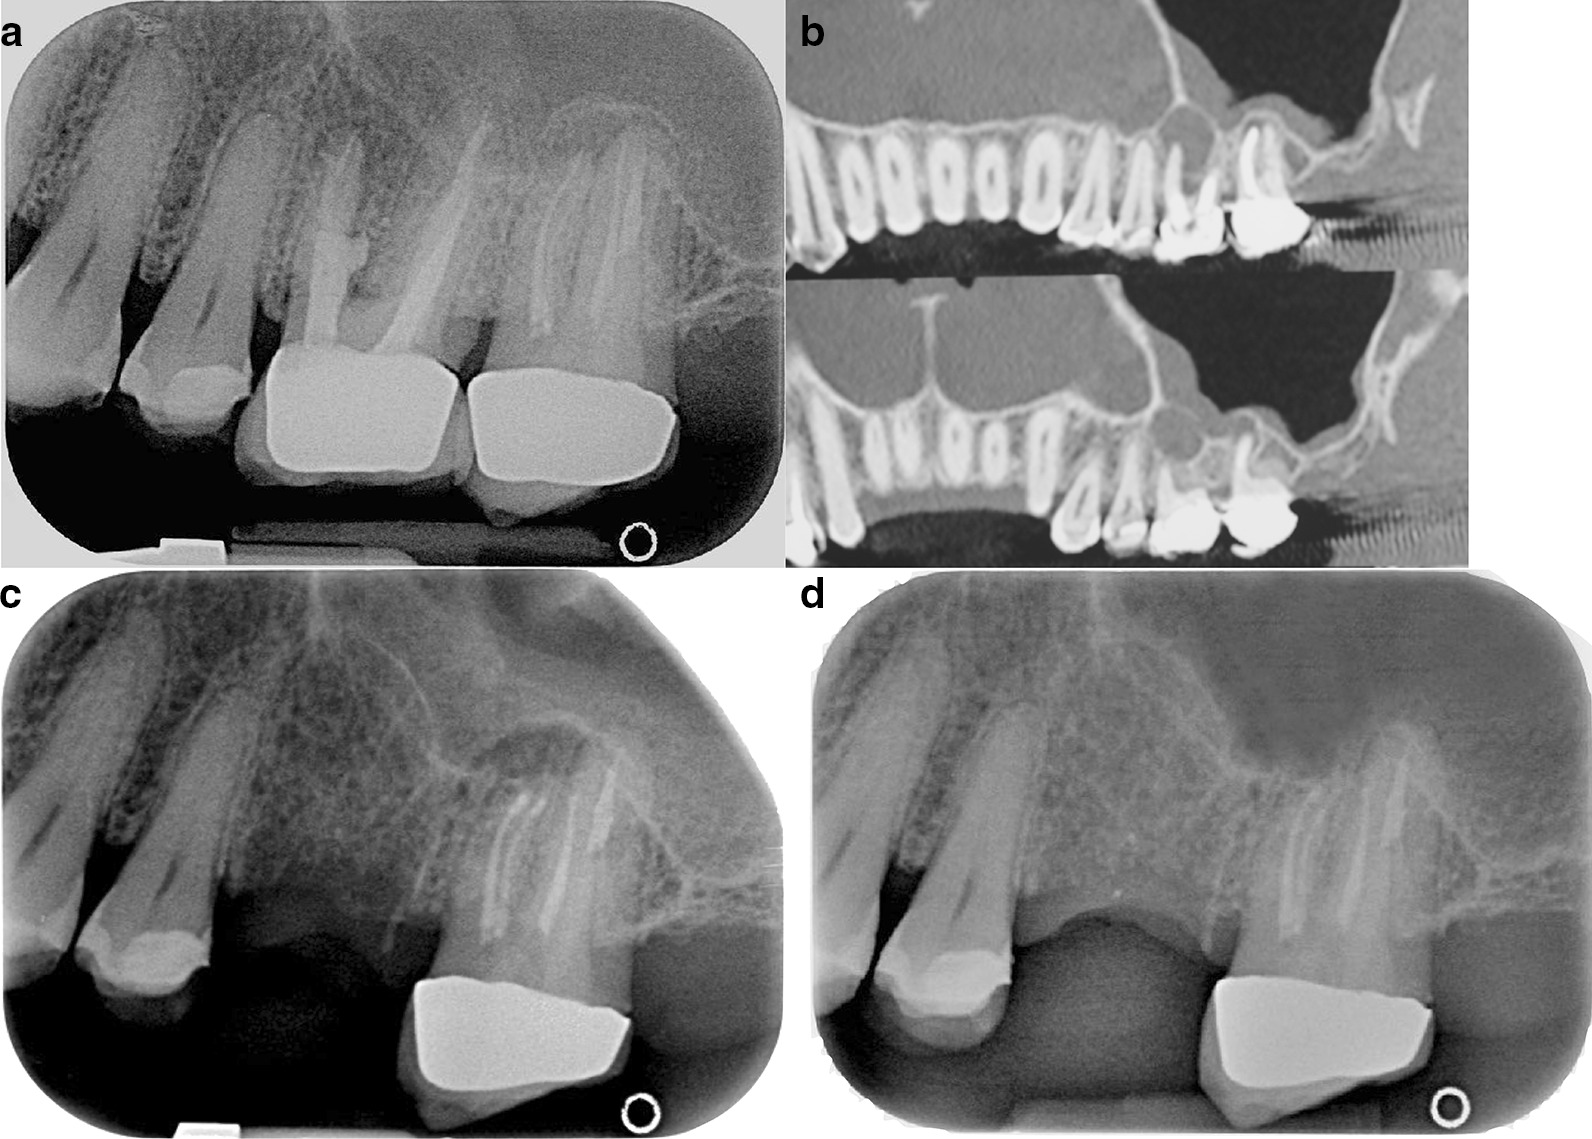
a Pre-operative periapical radiograph showing perforation on MB of 2.6 and a 2.7 periapical lesion of MB and DB. Classified: 2.6: R2-L3 and 2.7: R3-L4. Tooth 2.7 was treated 21 months ago and subsequently retreated 12 months ago. It is evident that it is difficult to distinguish exactly which roots are affected by tooth pathology 2.7 using a 2D image. b Pre-operative CBCT showing a different classification. 2.6 Classified R3-L4 and 2.7 classified R4 and L4. c Periapical radiograph at 6 months follow-up. After Microsurgical Endodontics on MB1 e MB2 and DB root of 2.7 showing an incomplete healing. Tooth 2.6 was extracted. d Periapical radiograph at 12 months follow-up. After Microsurgical Endodontics on MB1 e MB2 and DB root of 2.7 showing a complete healing. Tooth 2.6 was extracted
